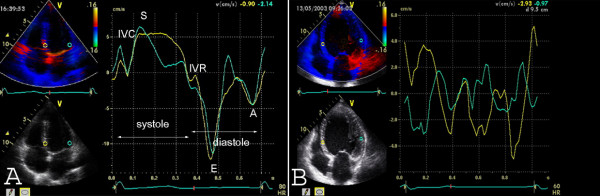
Tissue Doppler velocity data for the quantification of asynchrony from apical four chamber view. Sample volumes are in the basal lateral and basal septal segment. A) Normal control patient. There is a synchronous myocardial velocity in the septal (=yellow) and the lateral (=green curve) segment. IVC = isovolumetric contraction, IVR = isovolumetric relaxation, S = peak systolic velocity; E = early diastolic filling, A = late (atrial) diastolic filling. B) There is asynchronous myocardial velocity in the septal (=yellow) and the lateral (=green curve) segment.
